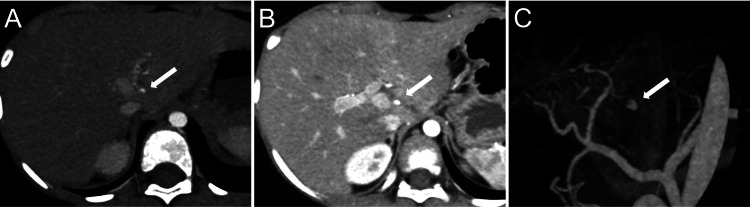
Follow-up contrast-enhanced computed tomography performed 10 days following the injury (A) Axial view of CECT in the early arterial phase, (B) axial view of CECT in the portal phase, and (C) three-dimensional CT angiography, showing a hepatic pseudoaneurysm (HPA) measuring 4 mm × 4 mm × 3 mm (white arrow).
